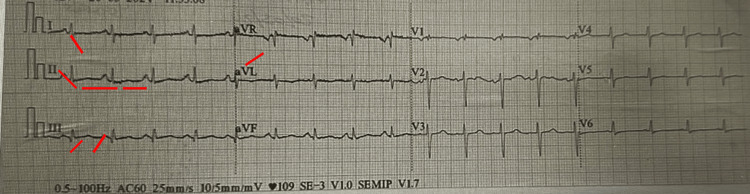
Twelve-lead echocardiogram. Sinus rhythm; heart rate (HR) 100 bpm; +60°; PR interval 0.16 s; QRS duration 0.08 s; S1Q3T3 pattern with incomplete right bundle branch block.
